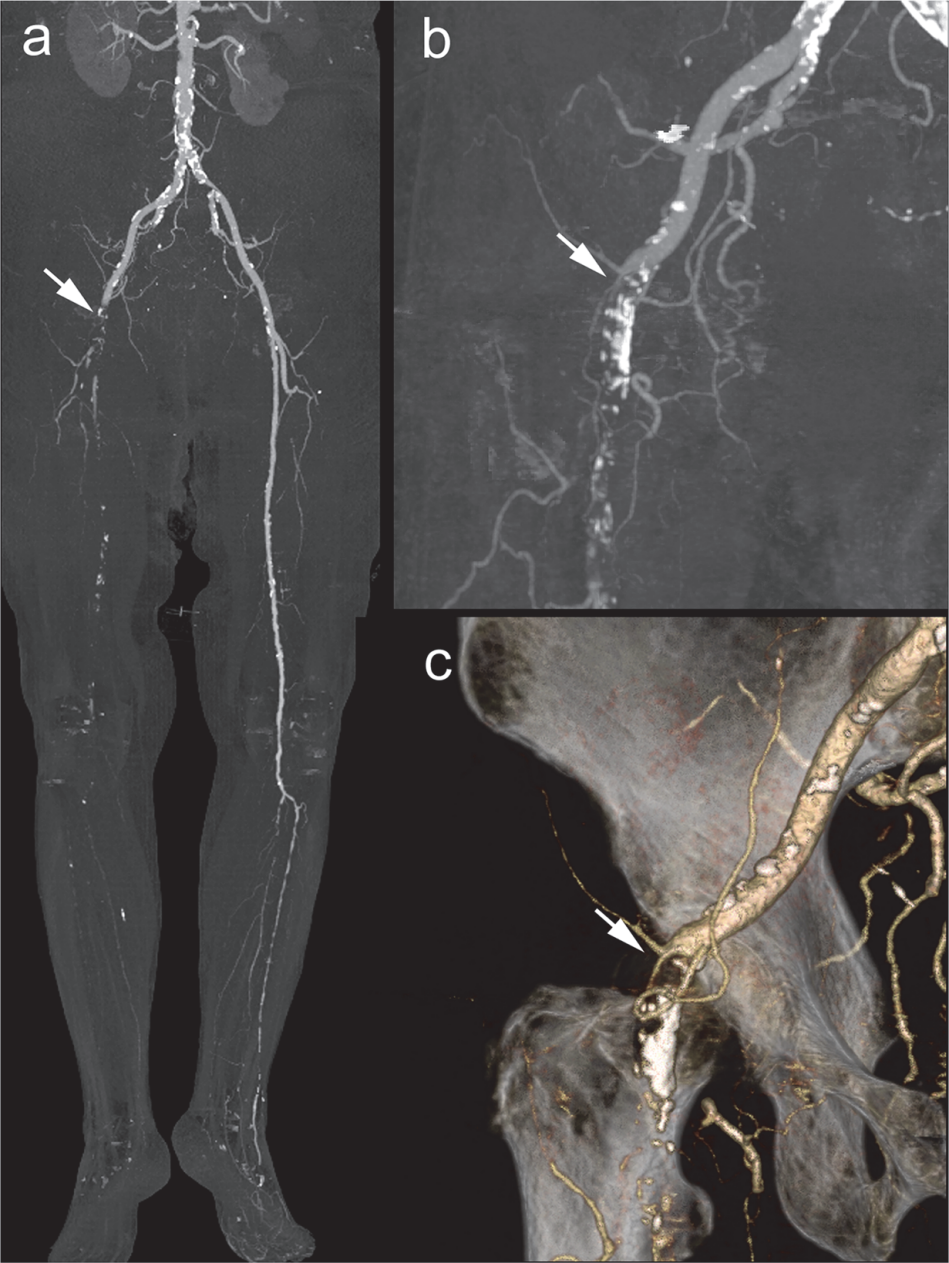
Example of a run-off CTA with sufficient diagnostic confidence despite non-diagnostic image quality. 84 y old female with acute onset of severe right leg pain. MIP (a,b) and volume rendering technique image (c) of run-off CTA revealed a thromboembolic occlusion with lack of arterial enhancement of the large arteries of the right leg from the external iliac artery (white arrow) down to the foot. Immediate surgical thrombectomy confirmed the finding of run-off CTA.
